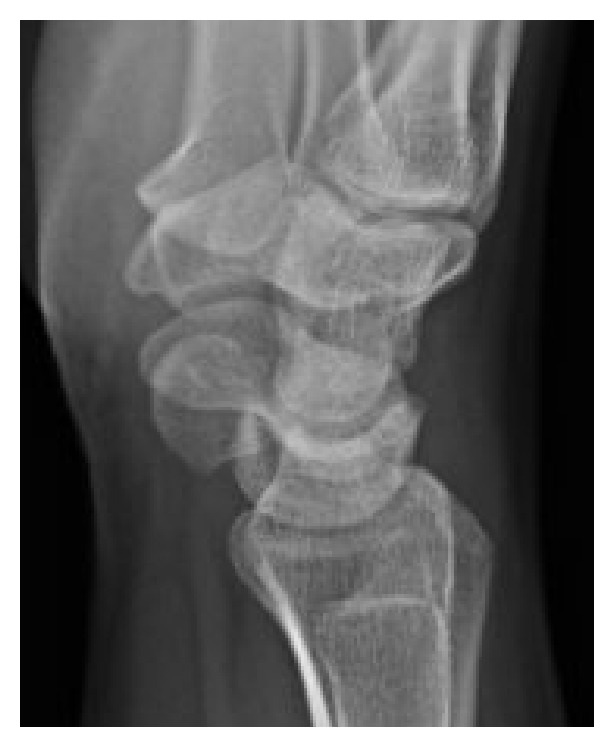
A lateral view of the wrist at the time of initial evaluation without radiographic abnormality of the scaphoid.
